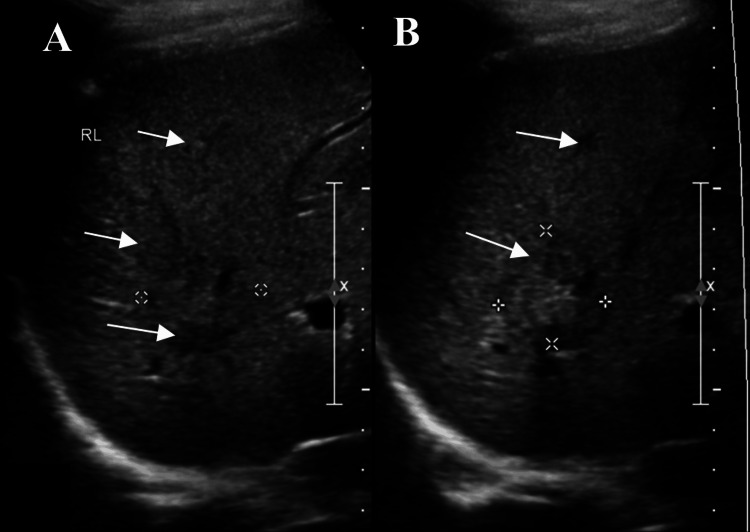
Ultrasound of the abdomen. Ultrasound images of the abdomen demonstrate multiple hepatic masses that require further assessment.
